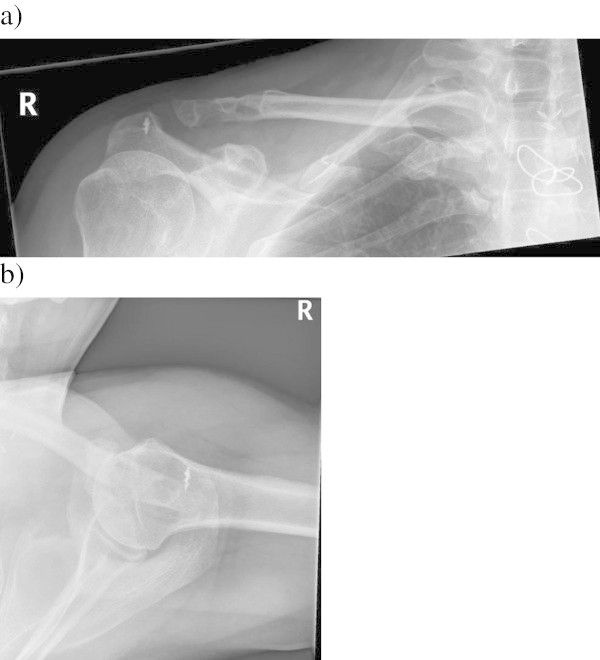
Anteroposterior (a) and axillary (b) radiographs of a patient after surgery for chronic AC joint dislocation. The injury was treated with autogenous semitendinosus and gracilis tendon grafts 5 years before. Notable tunnel widening has appeared in both drill holes. Erosion of the coracoid process is also visible.
